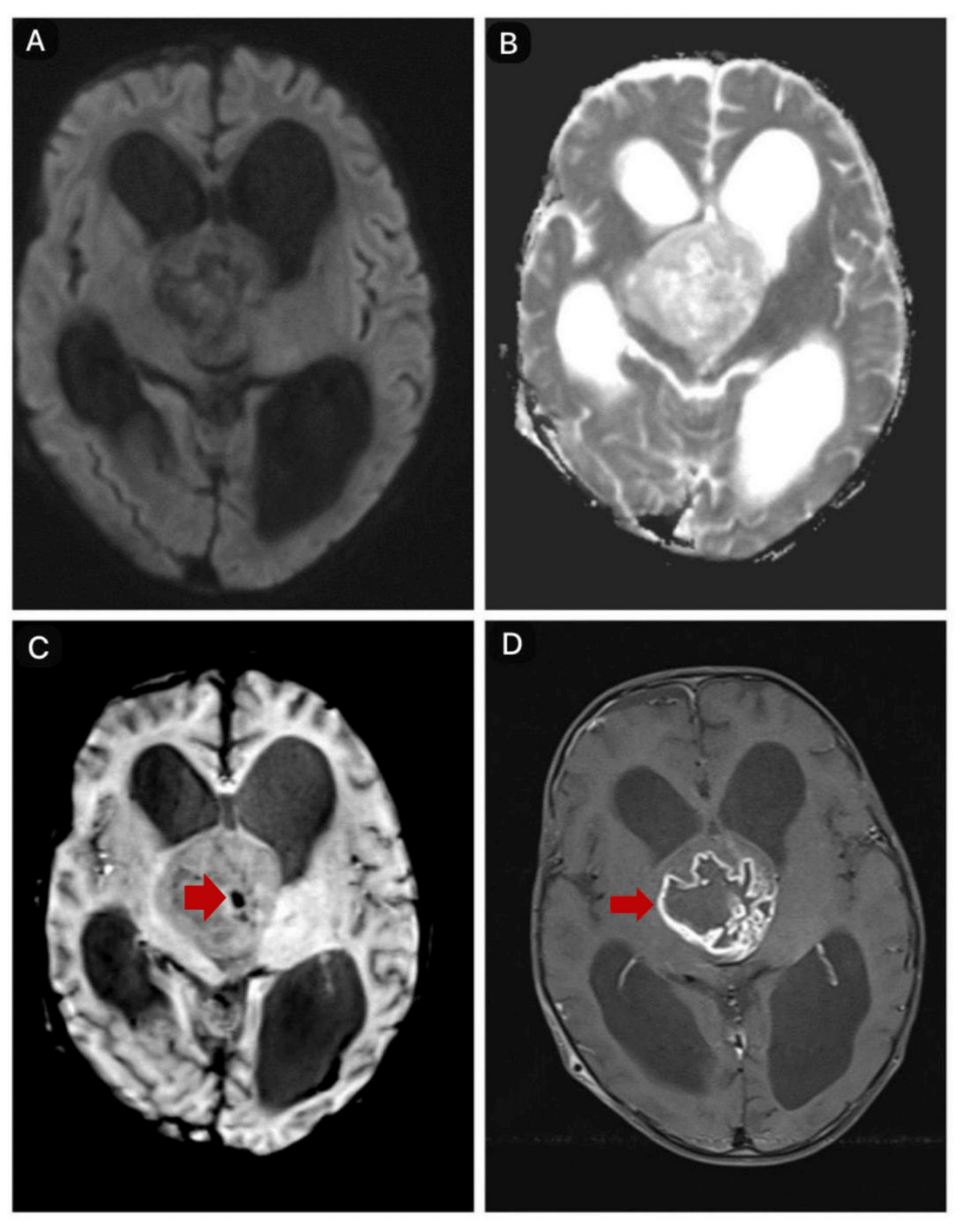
MRI brain A and B) Diffusion-weighted imaging showing no diffusion restriction and corresponding ADC image. C) Susceptibility-weighted imaging showing a signal dropout (indicated by a red arrow) suggestive of hemorrhage. D) T1 fat-saturated post-contrast image showing heterogeneous peripheral enhancement (indicated by a red arrow). MRI: magnetic resonance imaging; ADC: apparent diffusion coefficient
